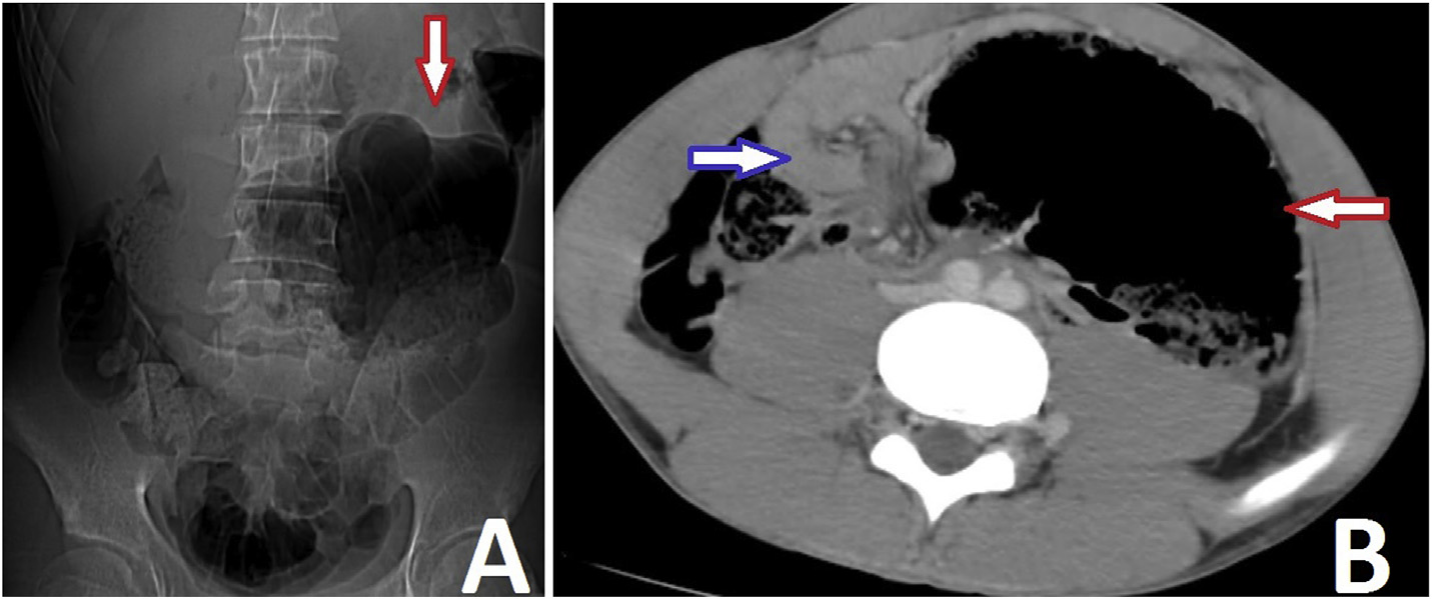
A: Plain radiography revealed a markedly air-distended bowel in the left abdomen, B: Abdominal CT scan showing coffee bean sign (red arrow) and whirl sign (blue arrow). (For interpretation of the references to colour in this figure legend, the reader is referred to the Web version of this article.)
